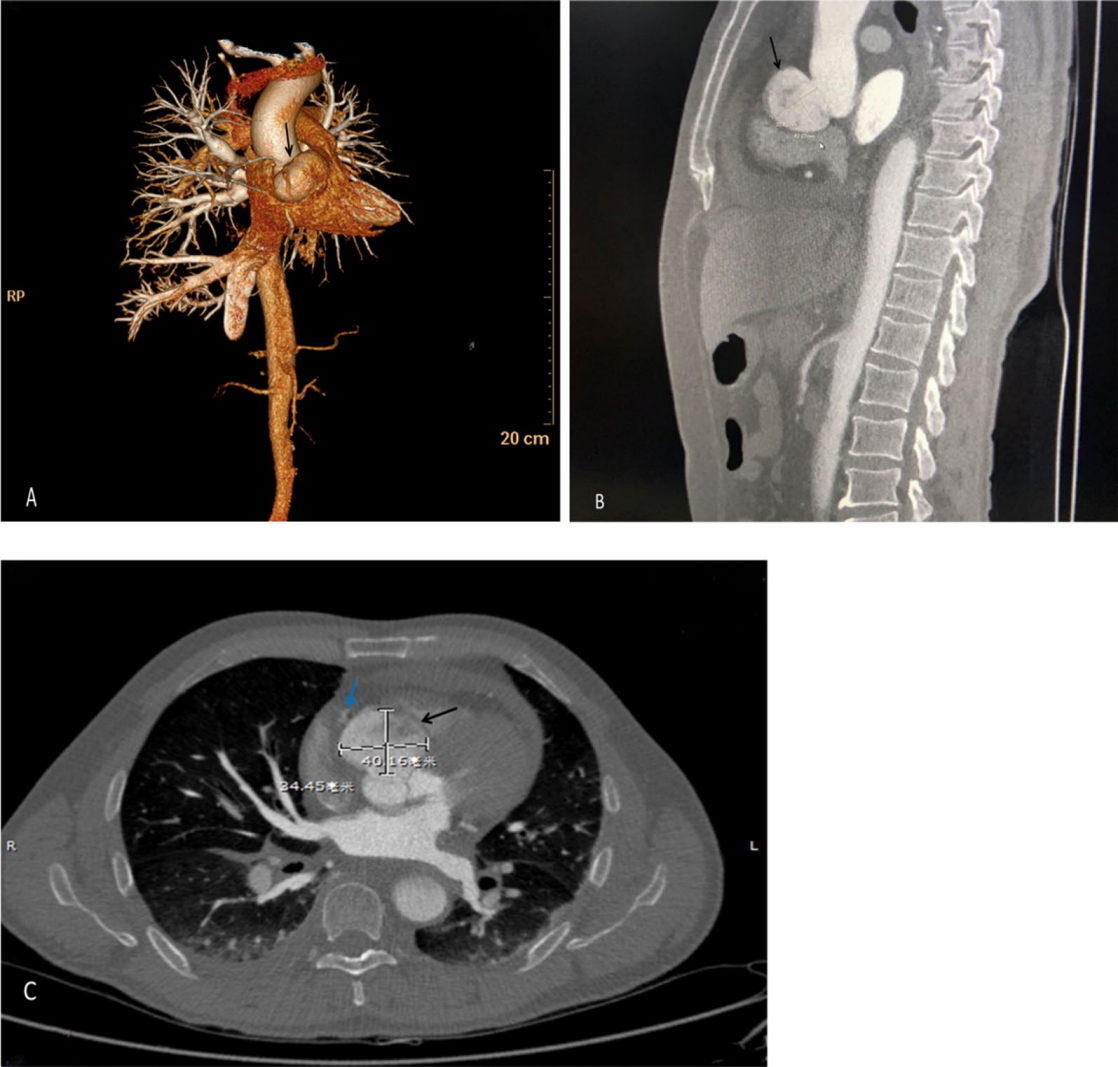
Aorta computed tomography angiography. Aorta computed tomography angiography showed a giant outward aortic right coronary sinus aneurysm (Panel A, B and C, black arrows) that was compressing on the ostium of the RCA (Panel C, blue arrow)
